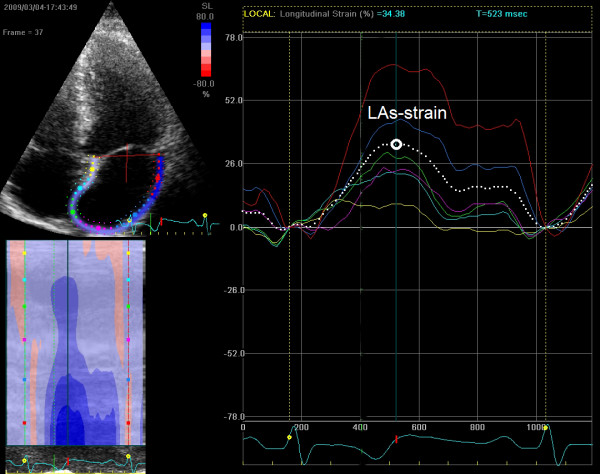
Measurement of LAs-strain on an image obtained from apical four-chamber view.*LAs-strain, peak left atrial strain during left ventricular systole.*
